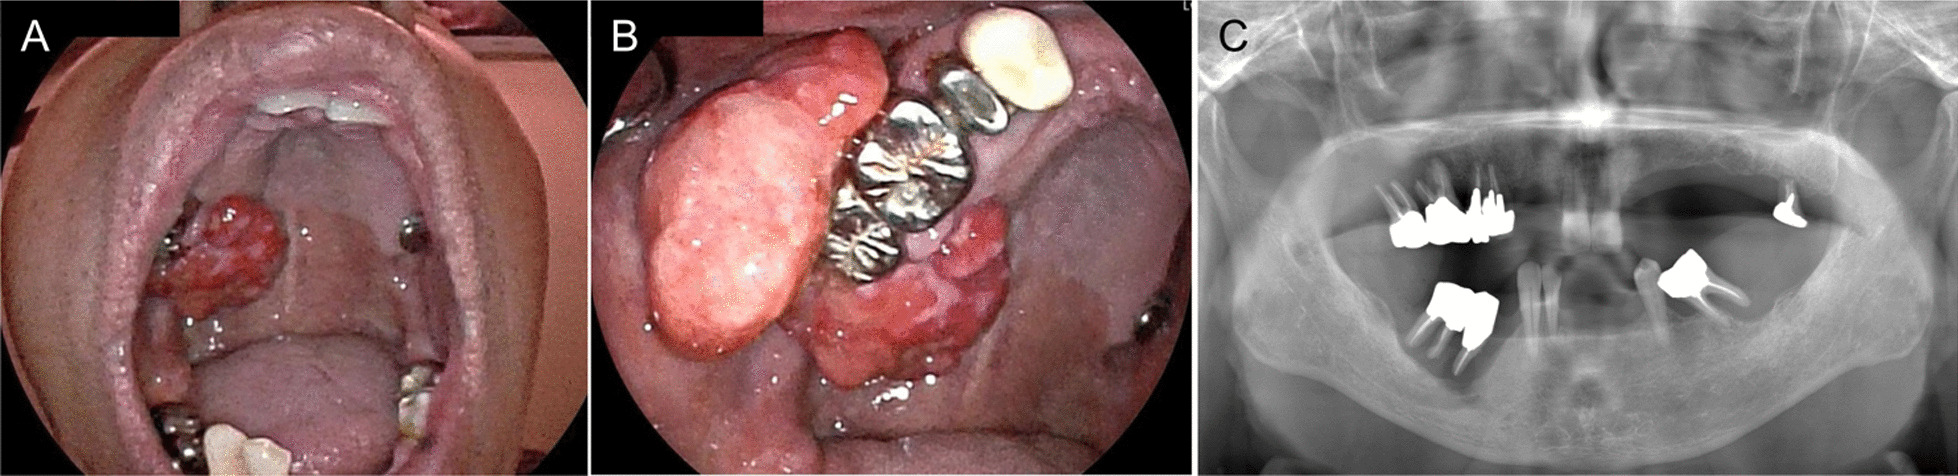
Intraoral and panorama-radiographic findings. A and B An elastic hard, seemingly well-defined, pedunculated exophytic tumor-like mass with a maximal diameter of approximately 60 mm was observed surrounding the right upper gingiva, including the right upper molars, extending medially from the hard palate, posteriorly to the retromolar trigone, and laterally to the buccal mucosa. C Orthopantomogram showing that all the remaining molars and premolars, including those surrounded by the right upper gingival mass, had severe alveolar bone resorption, indicating severe chronic periodontitis
